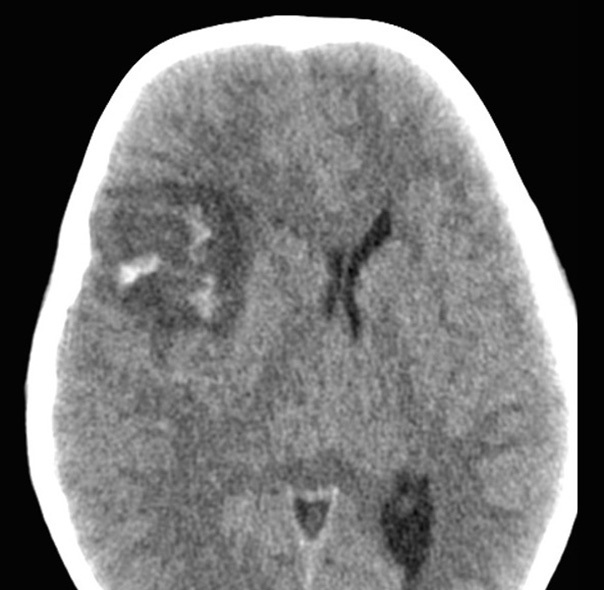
Axial CT soft tissue image showing a contusion injury in right frontal brain parenchyma and midline shift towards the contralateral side.
